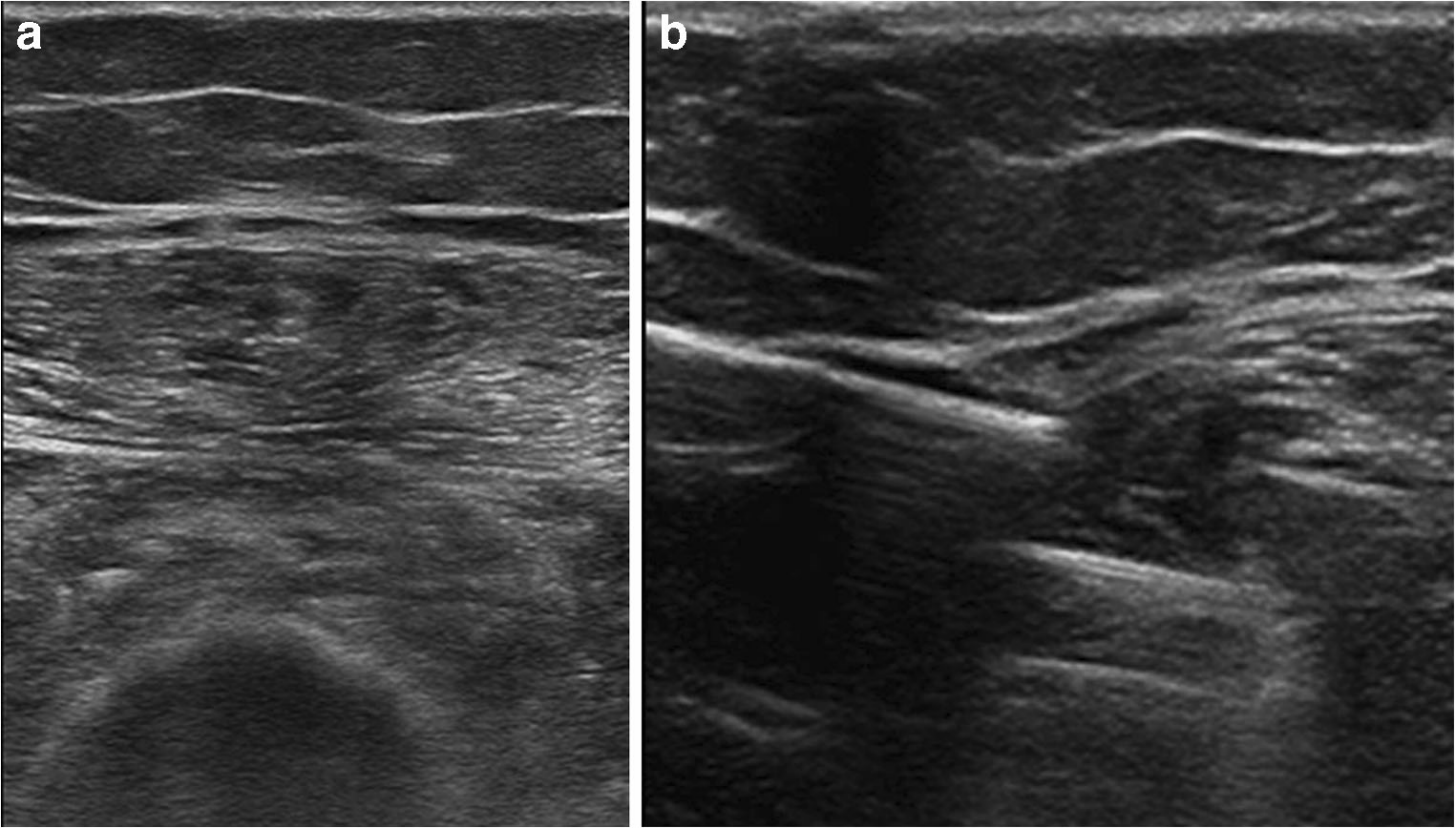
a Ultrasound of the leg demonstrates an expanded, hyperechoic semimembranosus muscle. b The muscle was targeted under real-time ultrasound guidance and biopsy samples retrieved utilizing a modified Bergström needle and suction technique. The image shows the gap for the biopsy and identifies the muscle fibres of the semimembranosus muscle being sampled
